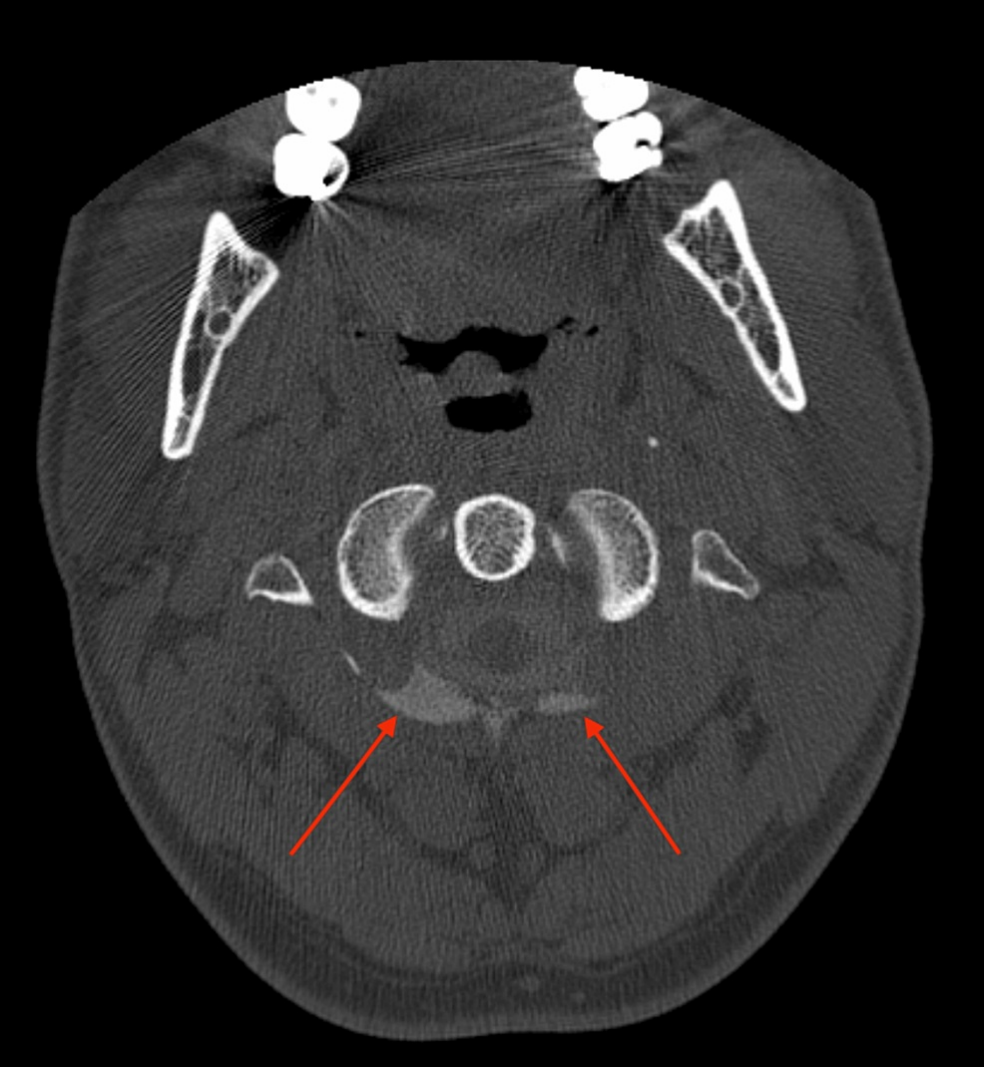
Axial C1-C2 level myelo-CT showing bilateral contrast outside the subarachnoid space (red arrows), as evidence of C1-C2 CSF fistulas.
